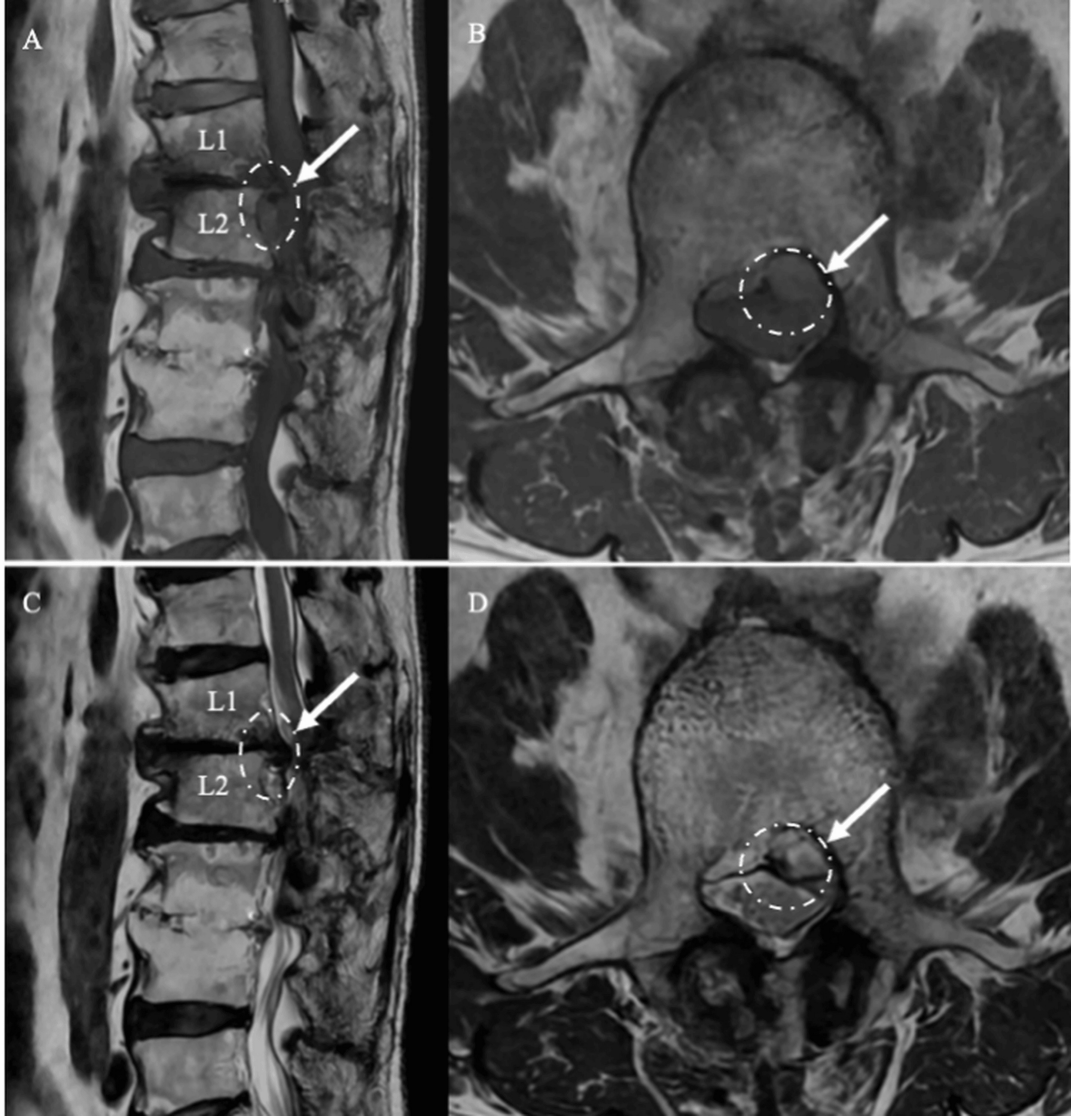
Postoperative MRI at Three Months Sagittal and axial T1-weighted (A, B) and T2-weighted (C, D) MRI scans obtained three months after surgery show a significant reduction in the gas component and sustained decompression of the nerve root at L1/2 *(white arrow *and dotted circle*)*.
